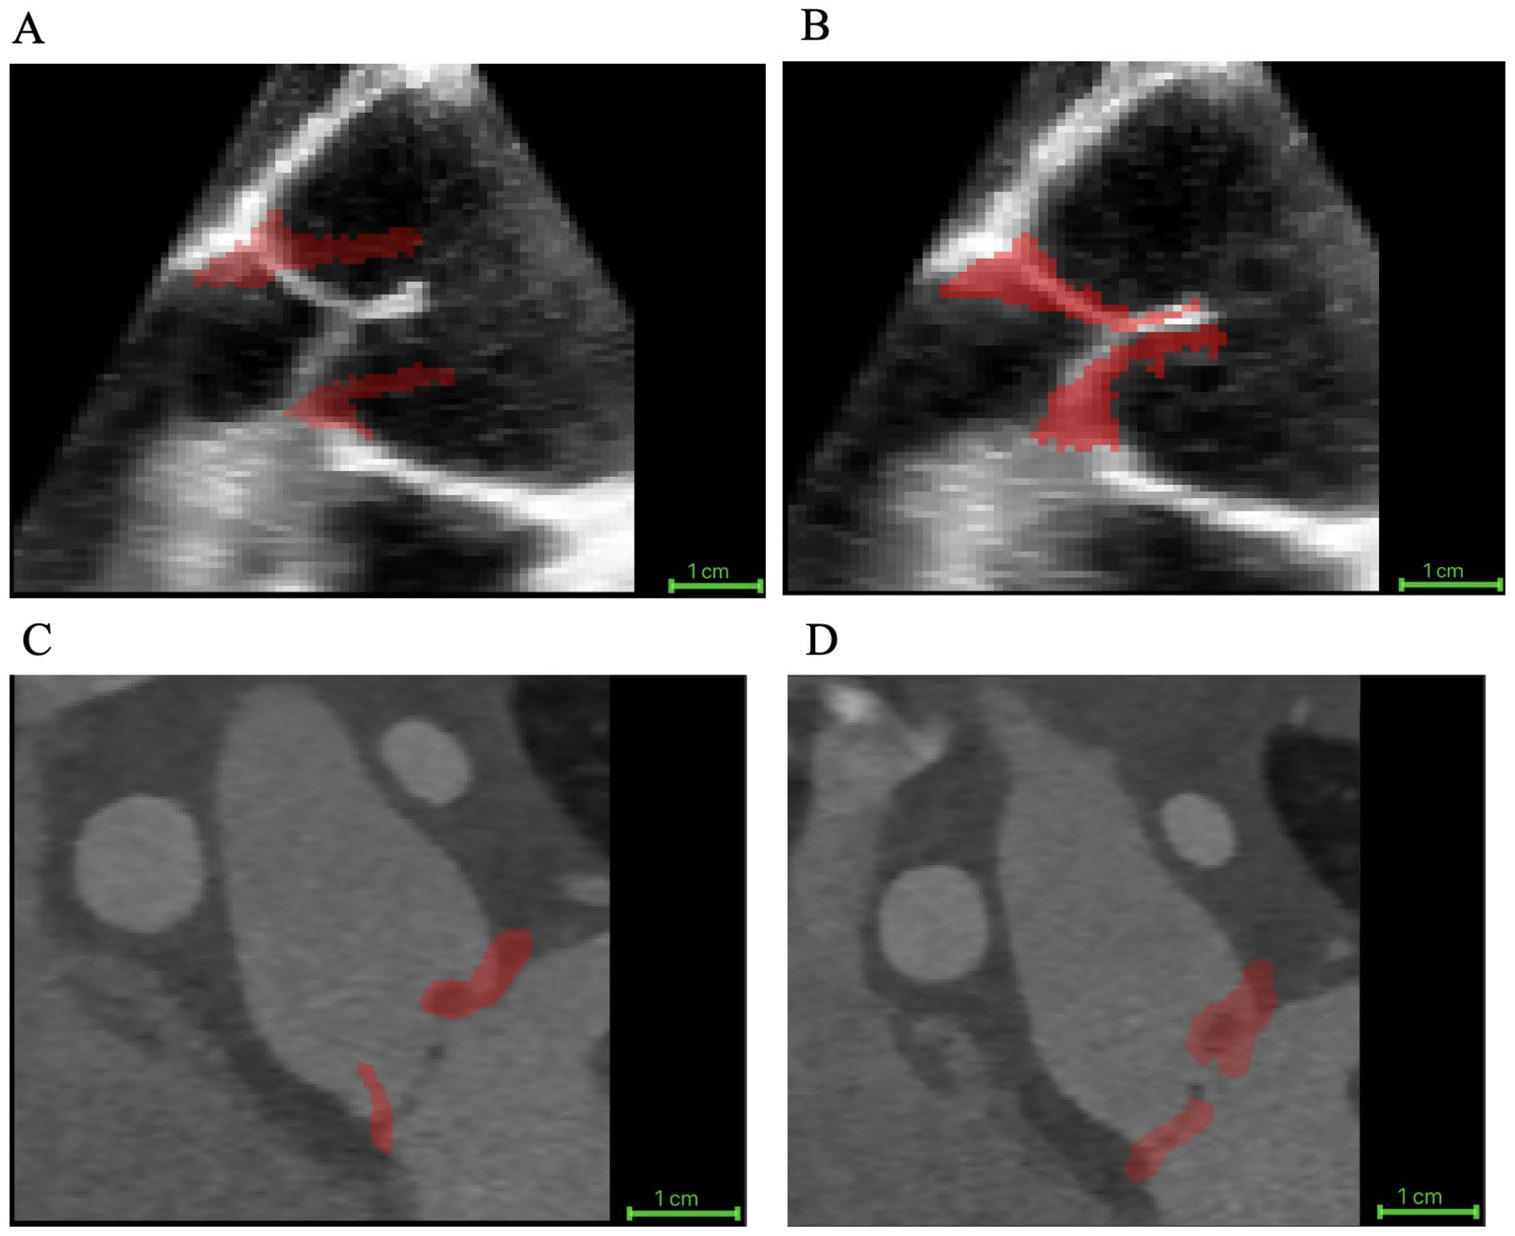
Example of image-based registration results for one TEE case and one CT case. The reconstructed aortic valve leaflets in the closed reference configuration are shown in red. A TEE with direct registration, B TEE with FEM-augmented registration, C CT with direct registration, and D CT with FEM-augmented registration
